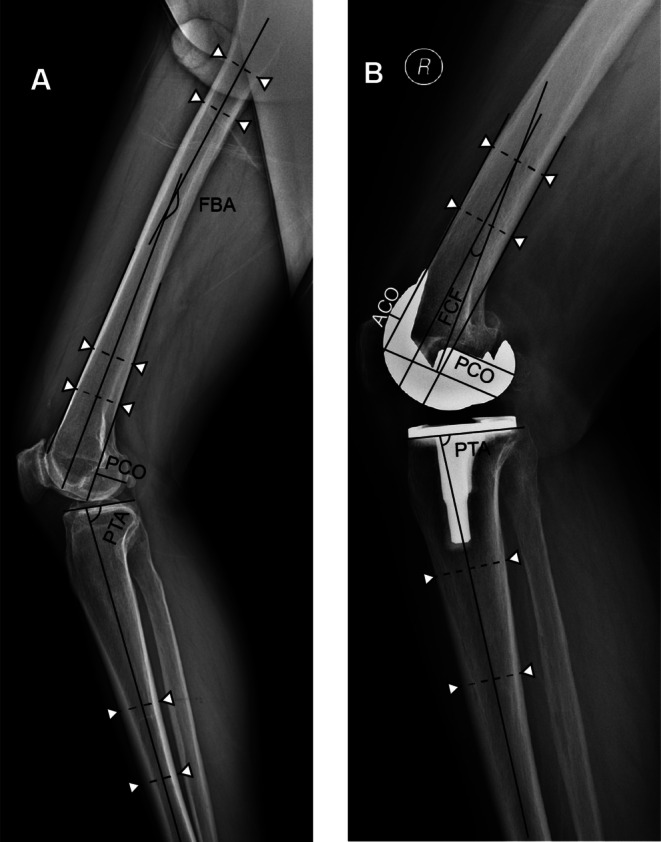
Sagittal variables in the radiological assessment. Figure 1 consists of two panels. Panel A shows a preoperative lateral knee radiograph, in which sagittal plane parameters are assessed. These include the posterior tibial slope, defined as the angle between a line connecting the anterior and posterior points on the tibial plateau and the sagittal mechanical axis; the thickness of the posterior femoral condyles, measured from a tangent line over the posterior femoral cortex; and the femoral bowing angle, defined as the angle between the distal and proximal femoral anatomical axes. Panel B displays a postoperative lateral knee radiograph, where the following sagittal alignment parameters of the knee prosthesis are evaluated: the posterior slope of the tibial component, the anterior condylar thickness, the posterior condylar thickness, and the flexion angle of the femoral component.
